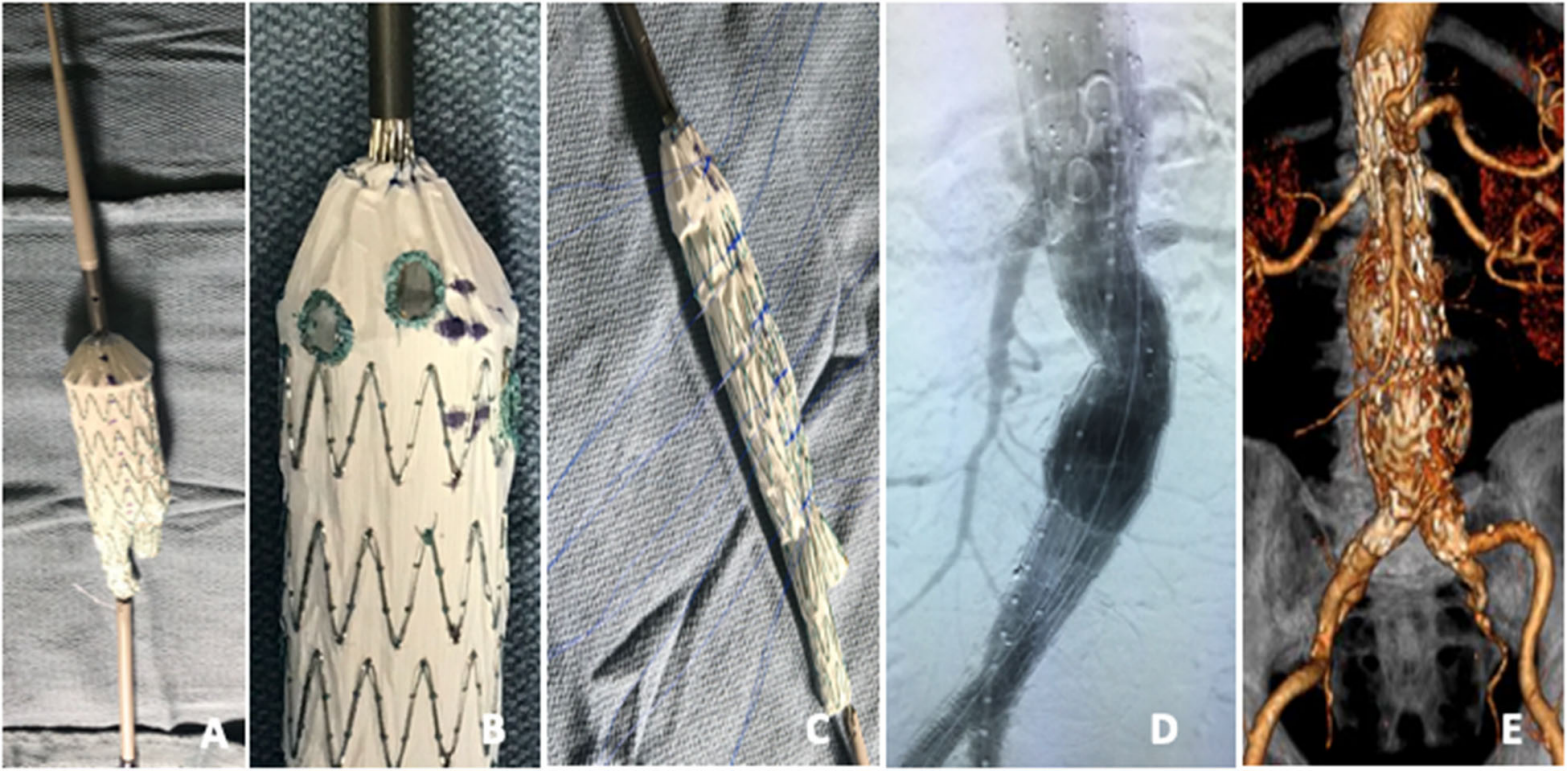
Steps involved in the modification of the Zenith Flex AAA bifurcated main body. The device is deployed on a sterile back table and one of the 3 nitinol wires is withdrawn from the inner cannula (A). Fenestrations are created using an ophthalmologic cautery and reinforced with a radiopaque snare using 4–0 Ethibond locking sutures (B). The nitinol wire is redirected through and through the fabric and the device is constrained every Z stent using the nitinol wire for support and two non-locking polypropylene loops prior to being resheathed (C). Completion angiography showing exclusion of the aneurysm and patency of all target vessels (D). 3D CTA obtained 24 months post-operatively showing continued patency of target vessels and aneurysm exclusion (E). Rationale for device selection: In a contained rupture JRAA amenable to 3 vessel PMEG, this two piece repair is ideal since, in case of frank rupture during implantation, the gate can be rapidly cannulated and contralateral limb placed, excluding the aneurysm prior to cannulating and bridging fenestrations. However, to avoid malalignment, we recommend bridging at least one fenestration (usually the SMA) prior to removing the diameter reducing tie. Provided sizing was accurate, one should still be able to cannulate and bridge renal artery fenestrations after the aneurysm is excluded
